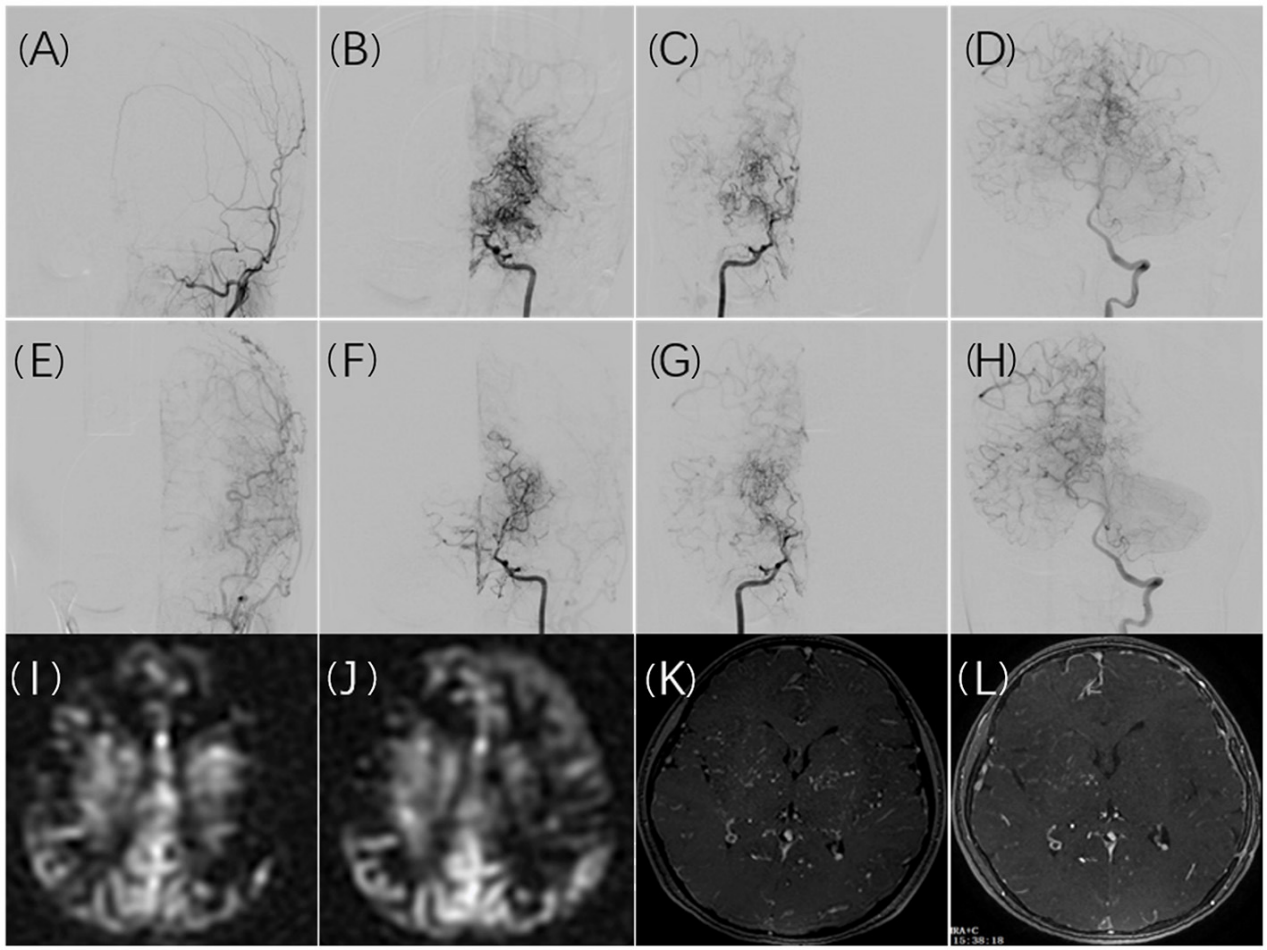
A 10-year-old girl presented with recurrent numbness of the right limbs. Cerebral angiography revealed bilateral moyamoya disease (B,C) without collateral anastomosis for the left ECA (A). Collateral compensation from the posterior circulation was noted (D). Six months after surgical revascularization, the left ECA angiogram demonstrated graft patency and good angiogenesis (E). Shrinkage of moyamoya vessels on both hemispheres was noted (F,G), and the left internal carotid artery compensated for the right hemisphere (F). The compensatory range of the posterior circulation shifted to the non-operated territory (H). The MRI ASL images showed hypoperfusion in the cerebral cortex (I). The hypoperfusion in the left cortex improved after surgical revascularization with a decrease in perfusion in the left basal ganglia (J). Ivy collateral vessels in contrast MRA decreased obviously in the left and right basal ganglia after surgical revascularization (K,L).
